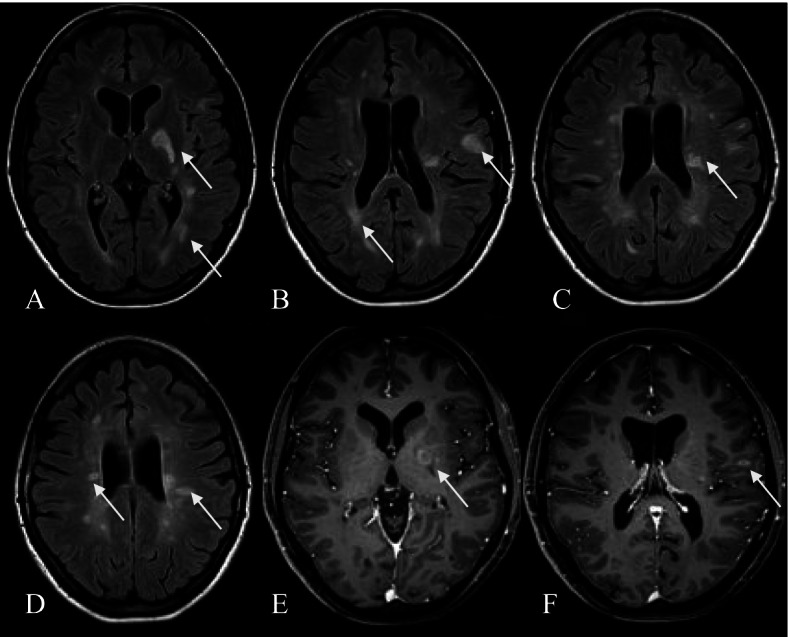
MRI of the patient (2020.09.20). A-D axial T2W FLAIR presents new hyperintense lesions (arrows). E–F T1W with contrast shows new gadolinium-enhancing lesions (arrows)
